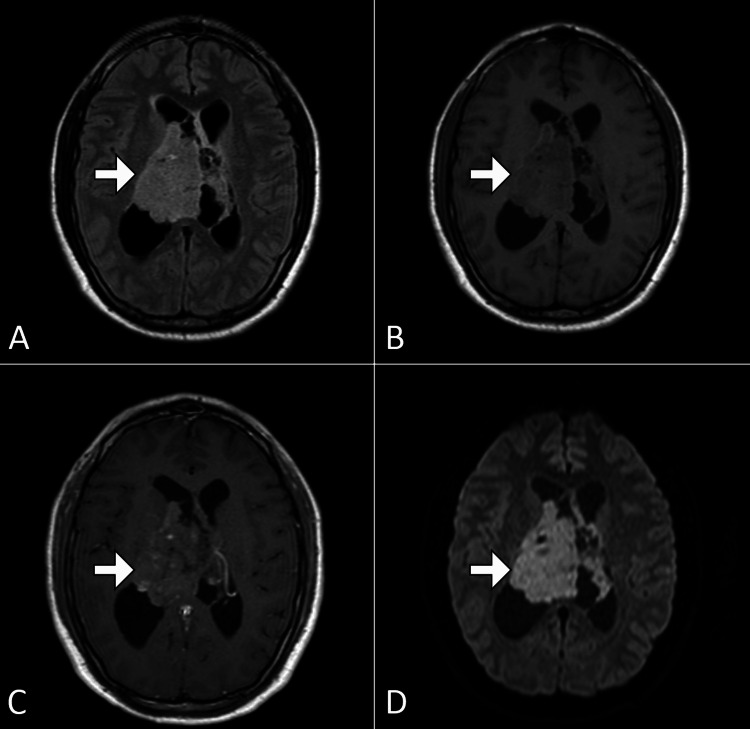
Axial MRI images of the brain Selected axial MRI images of the brain: FLAIR (A), T1-weighted (B), post-contrast T1-weighted (C), and DWI (D). The images reveal an ill-defined lesion (arrow) within the right lateral ventricle, centered on the septum pellucidum, causing obstructive hydrocephalus. MRI, magnetic resonance imaging; FLAIR, fluid-attenuated inversion recovery; DWI, diffusion-weighted imaging
